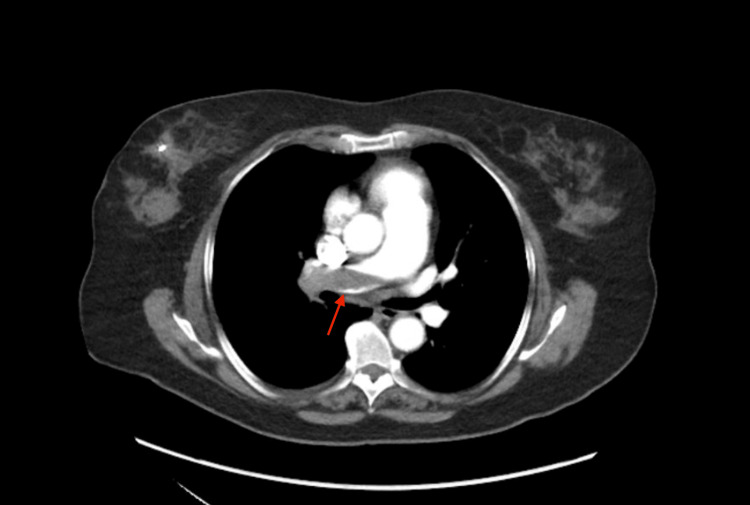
Initial CT Angiography Demonstrating Pulmonary Embolism A large pulmonary embolus in the right pulmonary artery is seen.
